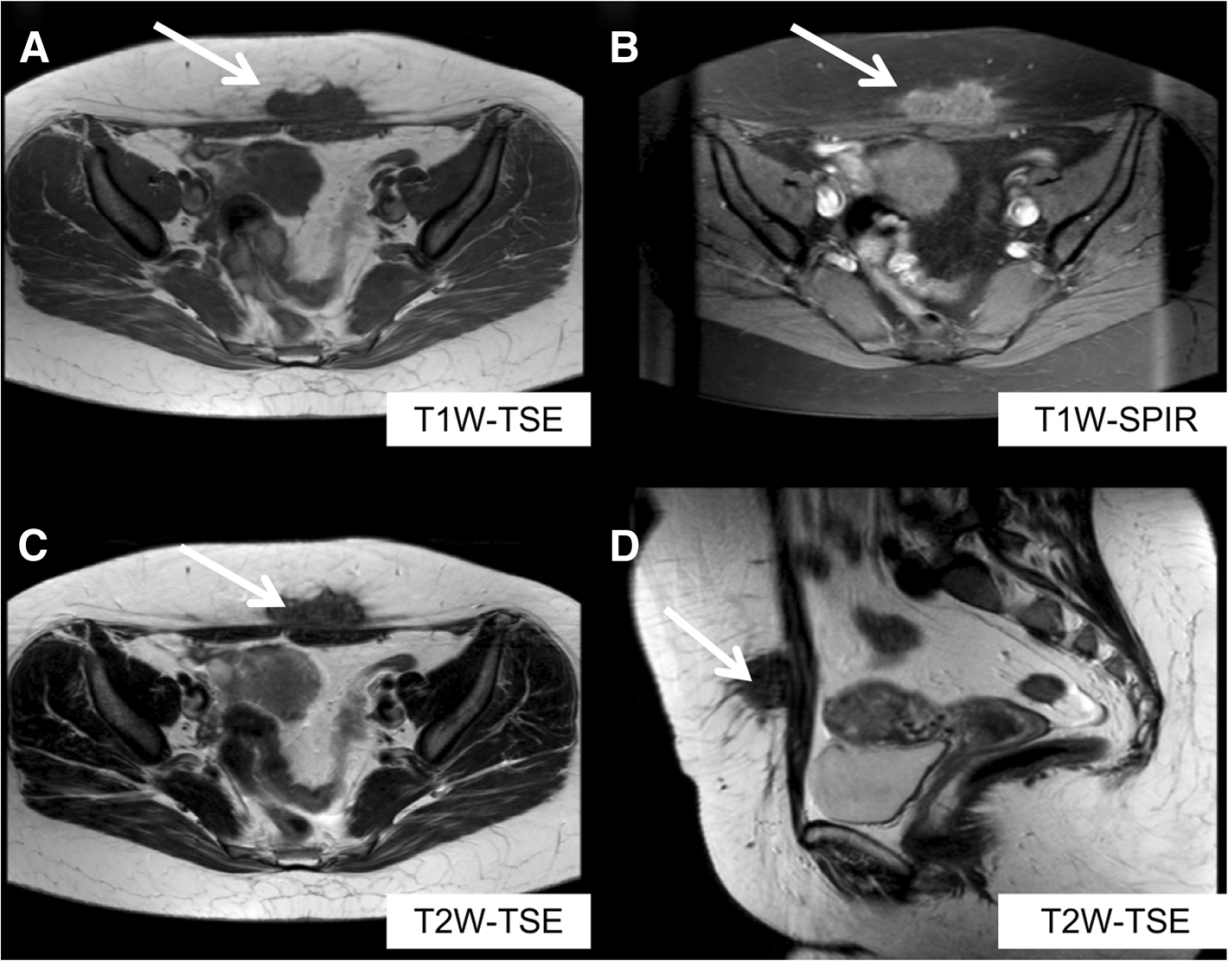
Abdominal wall endometriosis: this is a case of a women with abdominal wall palpable mass within incisions after cesarean delivery. MR imaging showed a solid nodule (white arrow) with low signal both on T1-TSE-WI (a) and T2-TSE-WI (axial, c and sagittal sections, d) with an iso-hyperintense signal on T1-SPIR WI (b)
